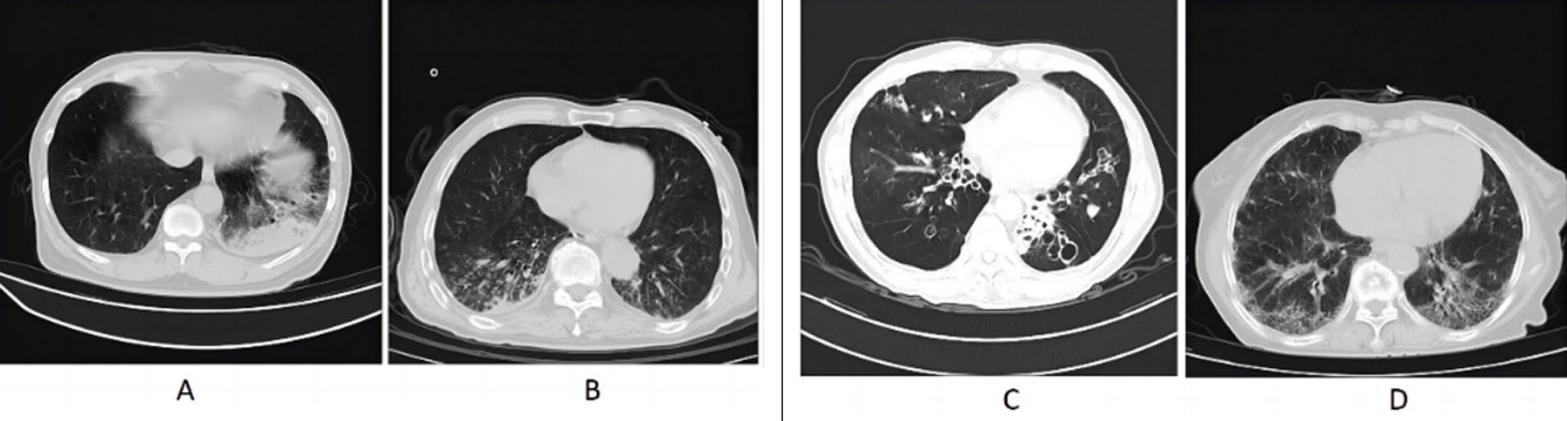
Chest CT images of patients with severe CAP. A: Male, 56 years old, with a large high-density shadow in the lower lobe of the left lung; B: Male, 62 years old, with multiple patchy and nodular high-density shadows in both lungs; C: Female, 63 years old, with extensive bronchiectasis and infection in both lungs; D: Female, 59 years old, with multiple patchy and grid like high-density shadows in both lungs.
